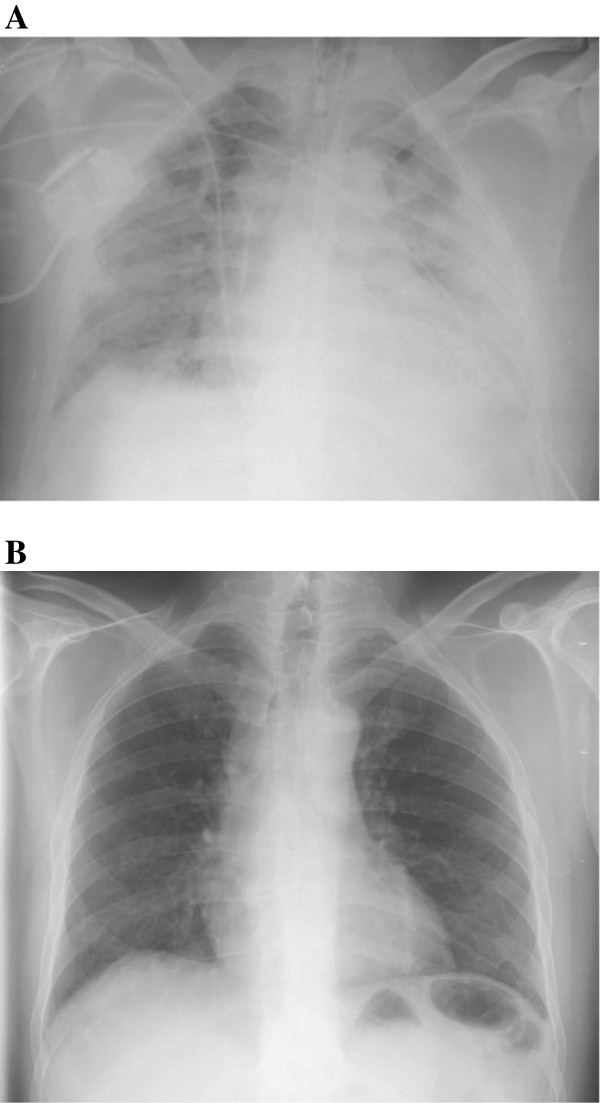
Chest X-ray before ECMO implantation (A) and before hospital discharge (B) in Patient 2. A. Bilateral parenchymal consolidation with air bronchograms, compatible with severe ARDS. B. Complete resolution of bilateral infiltrates.
